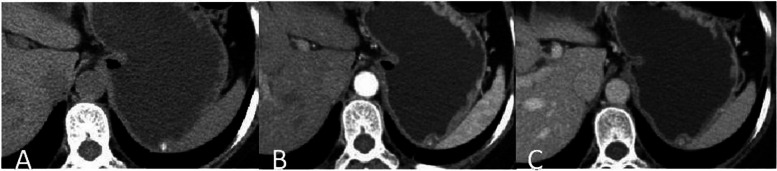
With a single stromal tumor in the gastric fundus. A Unenhanced CT scan showed nodular calcifications in the gastric fundus muscularis with no apparent soft tissue around them. CT scan in the arterial phase (B) and portal vein phase (C) showed no heterogeneous enhancement around the lesion, and a bulge in the adjacent mucosal layer
